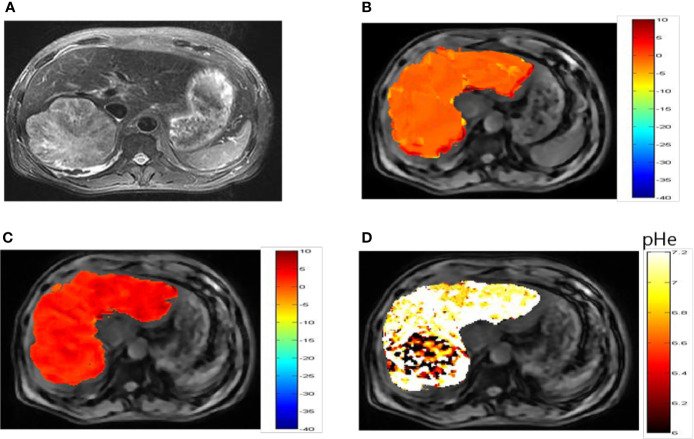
pH_e_ values of hepatic carcinoma using dual-power CEST MRI. (A) A representative image of a patient with hepatic carcinoma. (B, C) are CEST maps of hepatic carcinoma at flip angles of 60 and 350°. (D) pH_e_ map for the tumor volume. The CEST signal for the tumor area differed from the corresponding color of the adjacent normal liver tissue, with a lower pH_e_ value in the tumor area than in normal tissue. CEST, chemical exchange saturation transfer; pHe, extracellular pH; MRI, magnetic resonance imaging.
